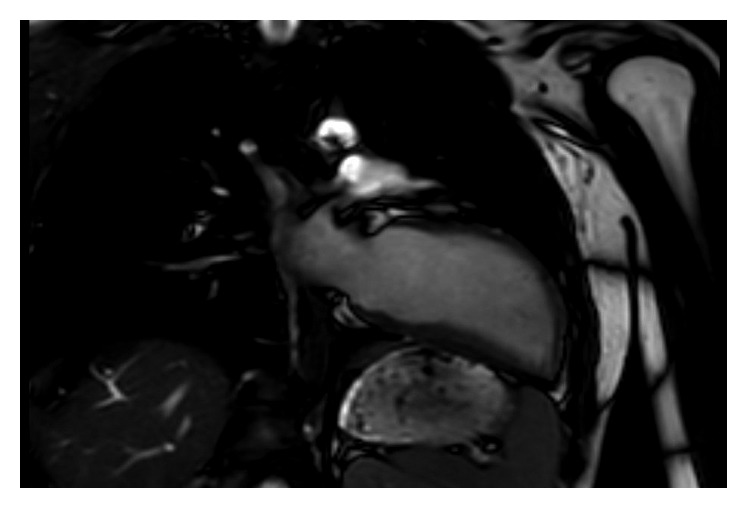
MRI cardiac showing typical teardrop shape of the heart with lung tissue between diaphragm and base of the heart.
